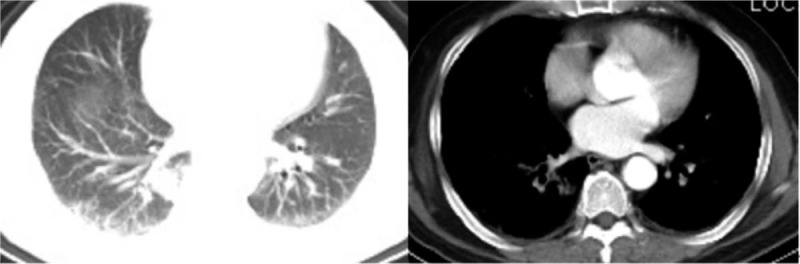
Preoperative chest computed tomography (CT) scan: Right lower lung nodules; interstitial pneumonia; and emphysema.
